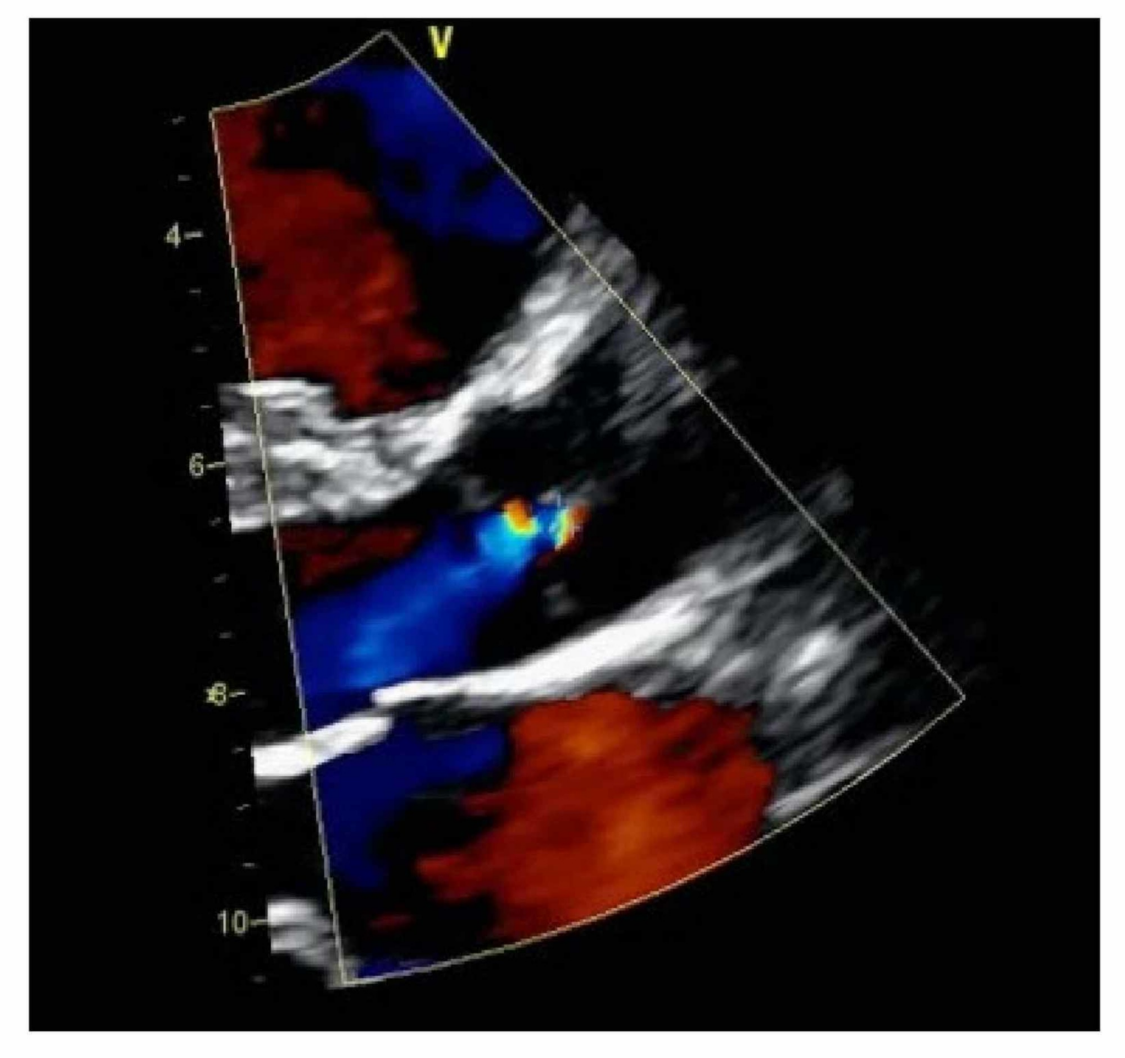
Transthoracic color Doppler echocardiogram showing mild aortic regurgitation
